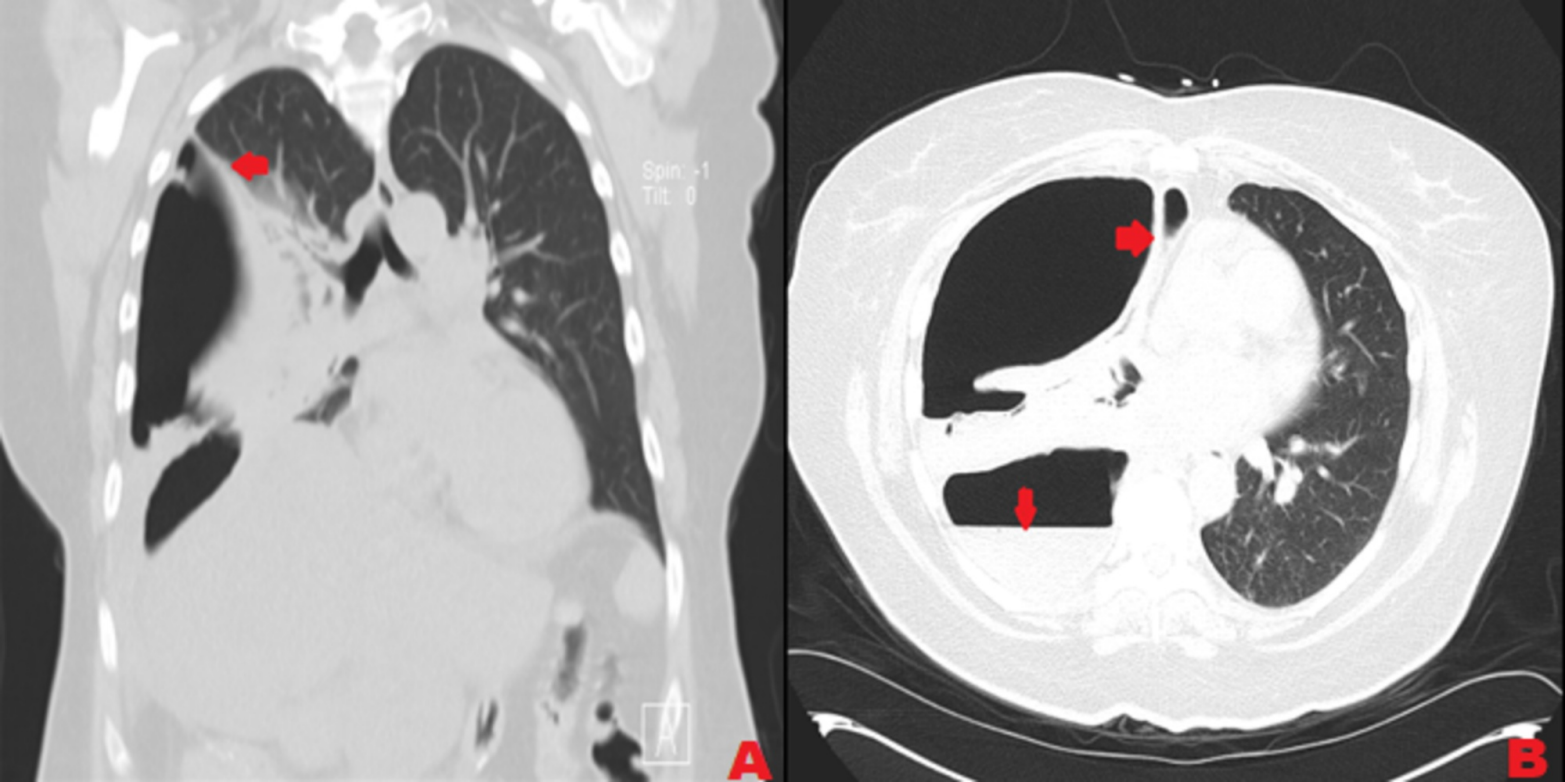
Computed tomography scan of the chest showing right-sided hydropneumothorax with almost complete collapse of the right lung A: Coronal view, B: Axial view
